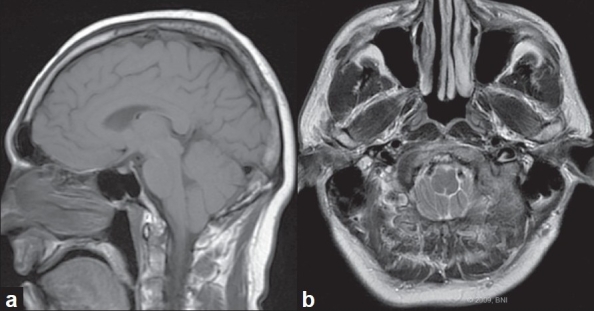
Preoperative (a) sagittal and (b) axial magnetic resonance images showing tonsillar herniation below the foramen magnum. [Used with permission from Barrown Neurological Institute]
